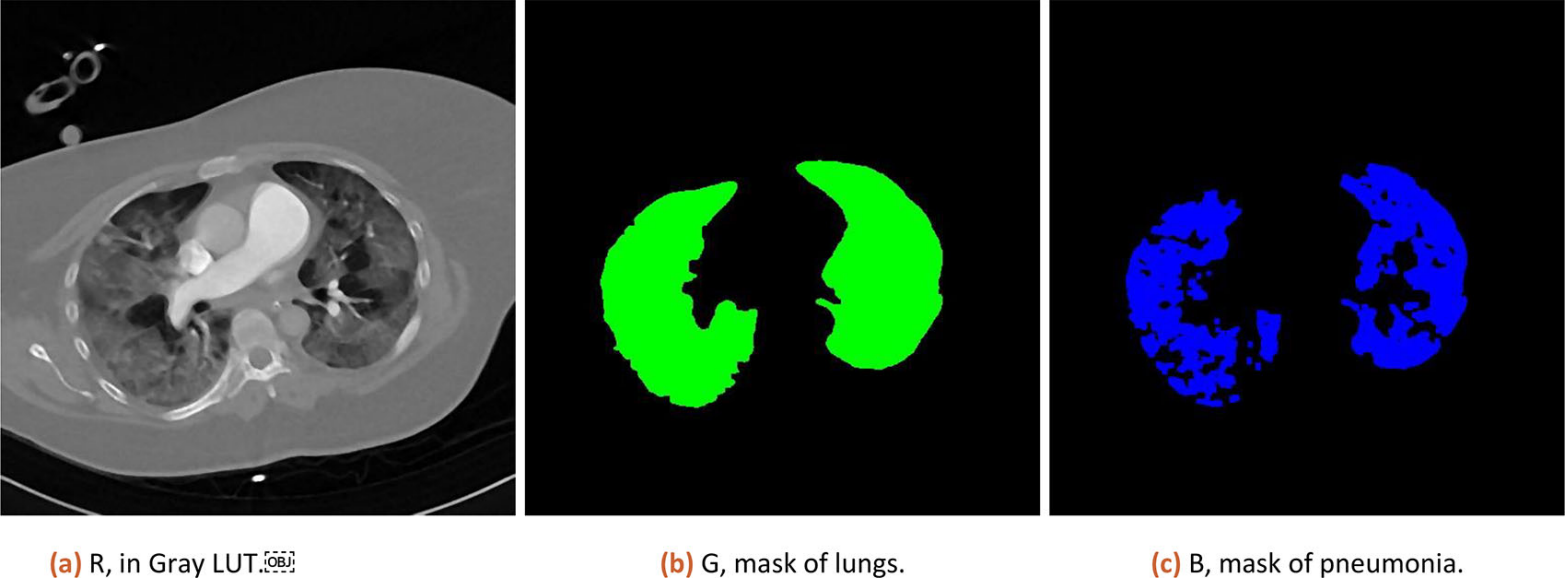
Result of analysis as RGB stack, where Red channel contains CT data, Green channel lung mask and Blue channel pneumonia mask.
